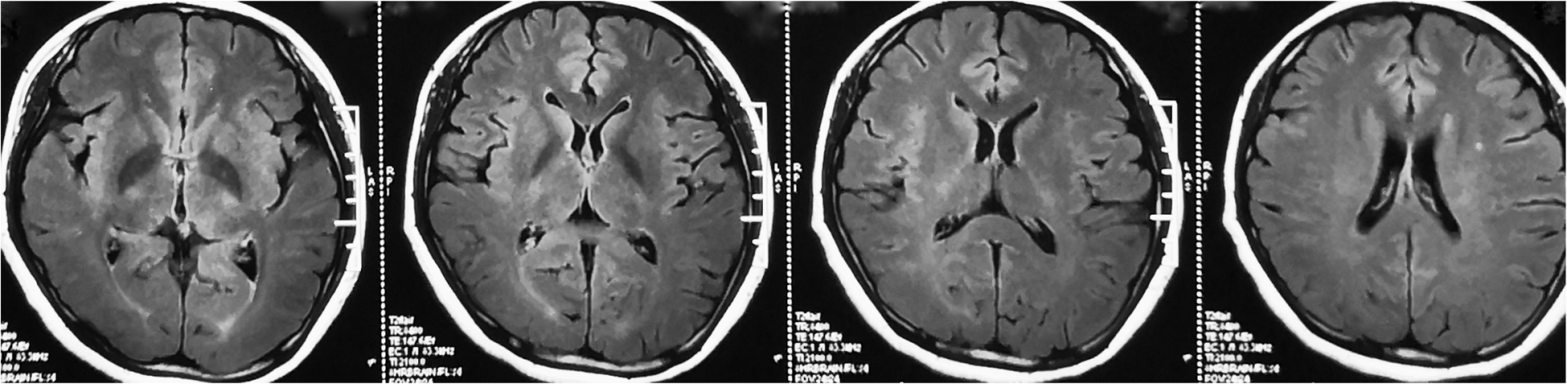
Axial T2 FLAIR sequence MRI showing bilateral cortical and subcortical hyperintensities
